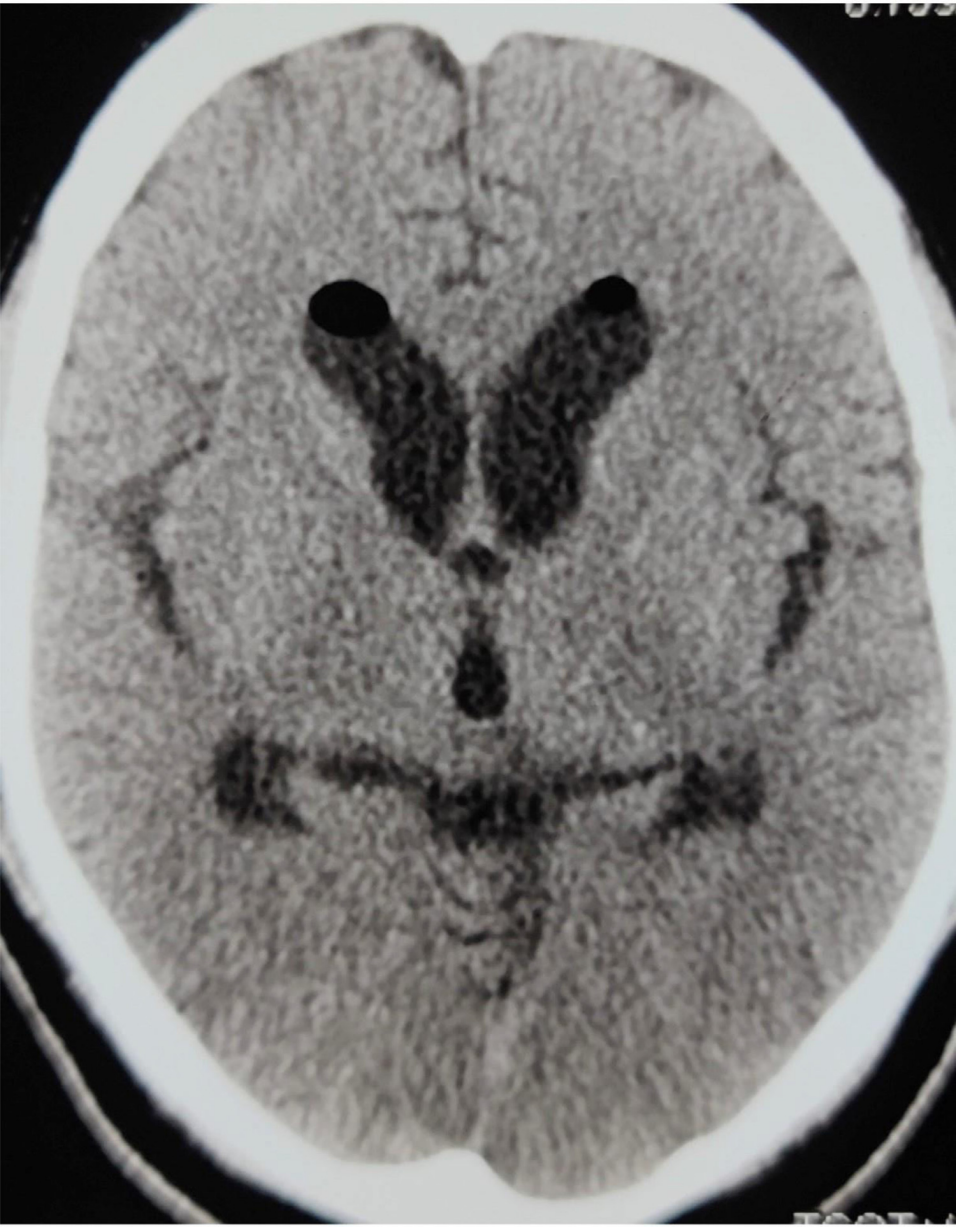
Plain CT head after removal of external ventricular drainage after 10 days. Hydrocephalus has completely resolved. Mild air is present in frontal horns of bilateral lateral ventricles.
